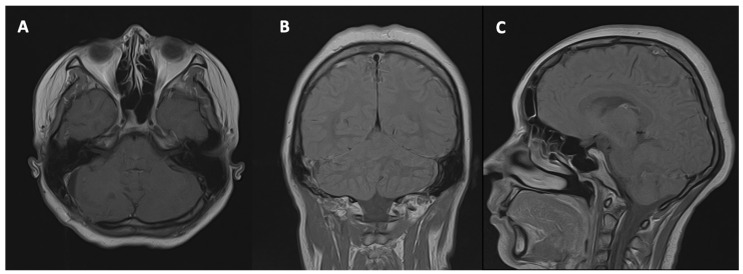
Follow-up MRI at 6 months after surgery. Axial T1-weighted imaging with contrast (A), coronal T1-weighted imaging with contrast (B), and sagittal T1-weighted imaging with contrast (C) demonstrated stable postoperative changes without evidence of residual or recurrent tumour. The posterior fossa structures were preserved, with normal configuration of the fourth ventricle and no signs of hydrocephalus or mass effect.
